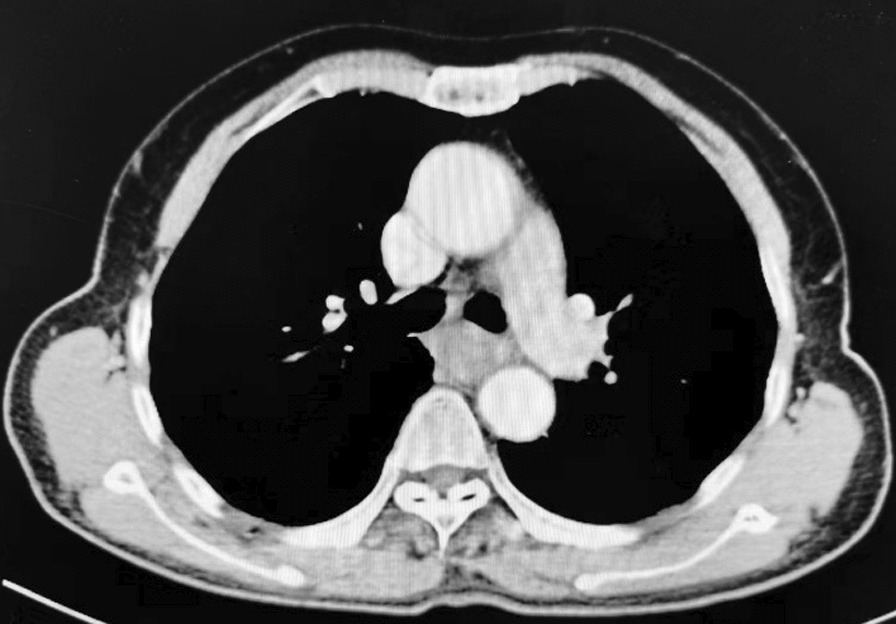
Enhanced CT of the chest
